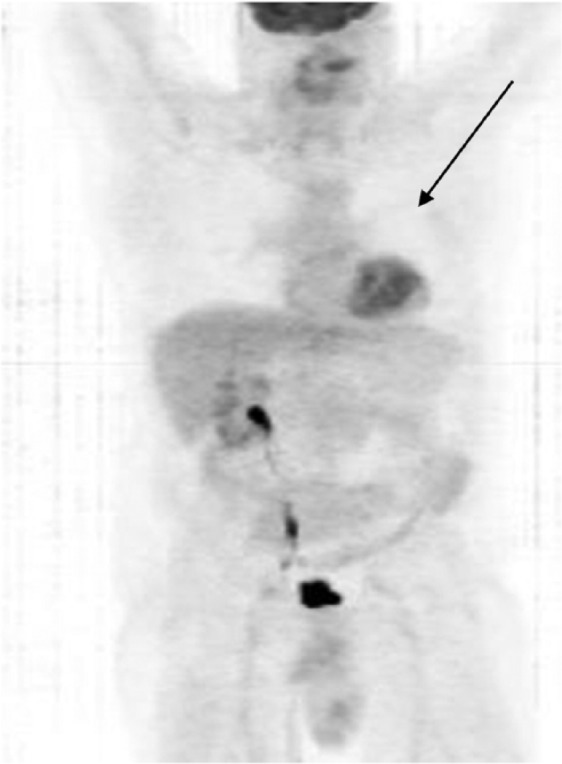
PET/CT scan, January 2014.
